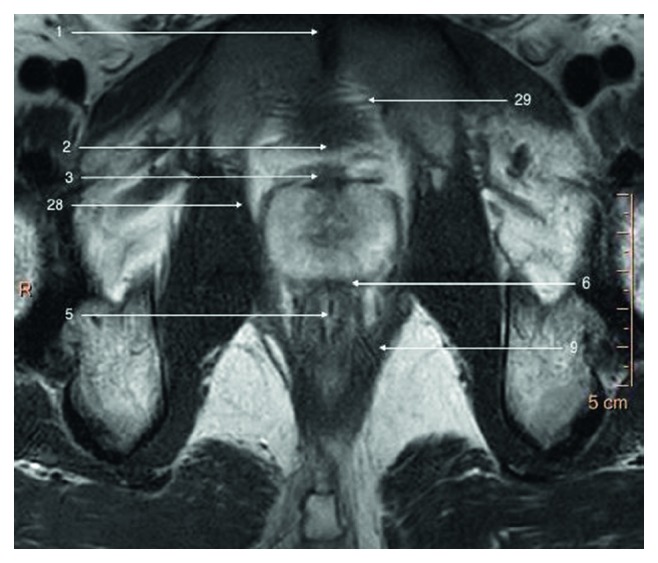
T2-weighted axial image of a prostate gland and surrounding structures.
